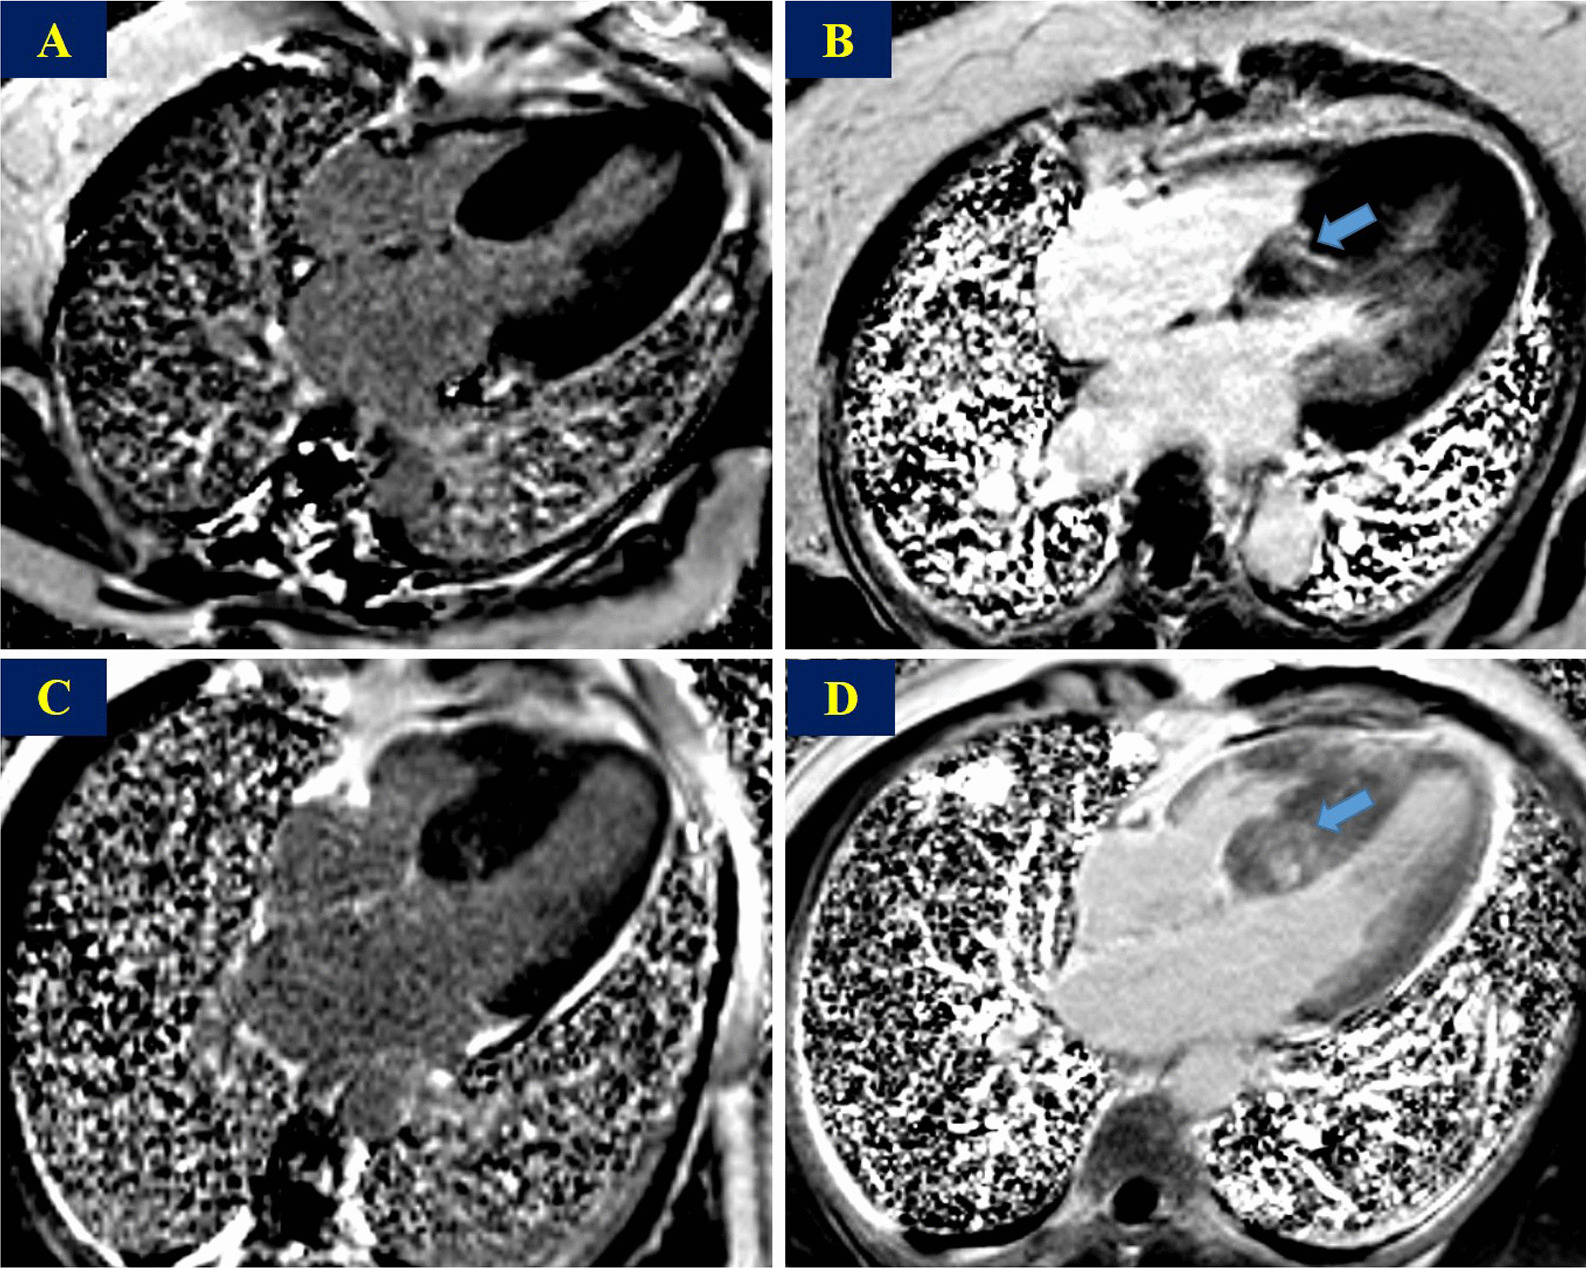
Contrast-enhanced magnetic resonance images in one female patient before ASA with an LVM index of 151.74 g/m^2^, an LVEDV index of 79.61 ml/m^2^ and a remote LGE of 13.52 g (A) and at follow-up after ASA with an LVM index of 141.26 g/m^2^, an LVEDV index of 84.61 ml/m^2^ and a remote LGE of 13.47 g (B) and in one male patient before ASA with an LVM index of 107.04 g/m^2^, an LVEDV index of 83.84 ml/m^2^ and a remote LGE of 9.16 g (C) and at follow-up after ASA with an LVM index of 90.59 g/m^2^, an LVEDV index of 89.09 ml/m^2^ and a remote LGE of 9.09 g (D). The blue arrows indicate the position of the alcohol ablation. *ASA* alcohol septal ablation, *LVM* left ventricular mass, *LVEDV* left ventricular end-diastolic volume, *LGE* late gadolinium enhancement
